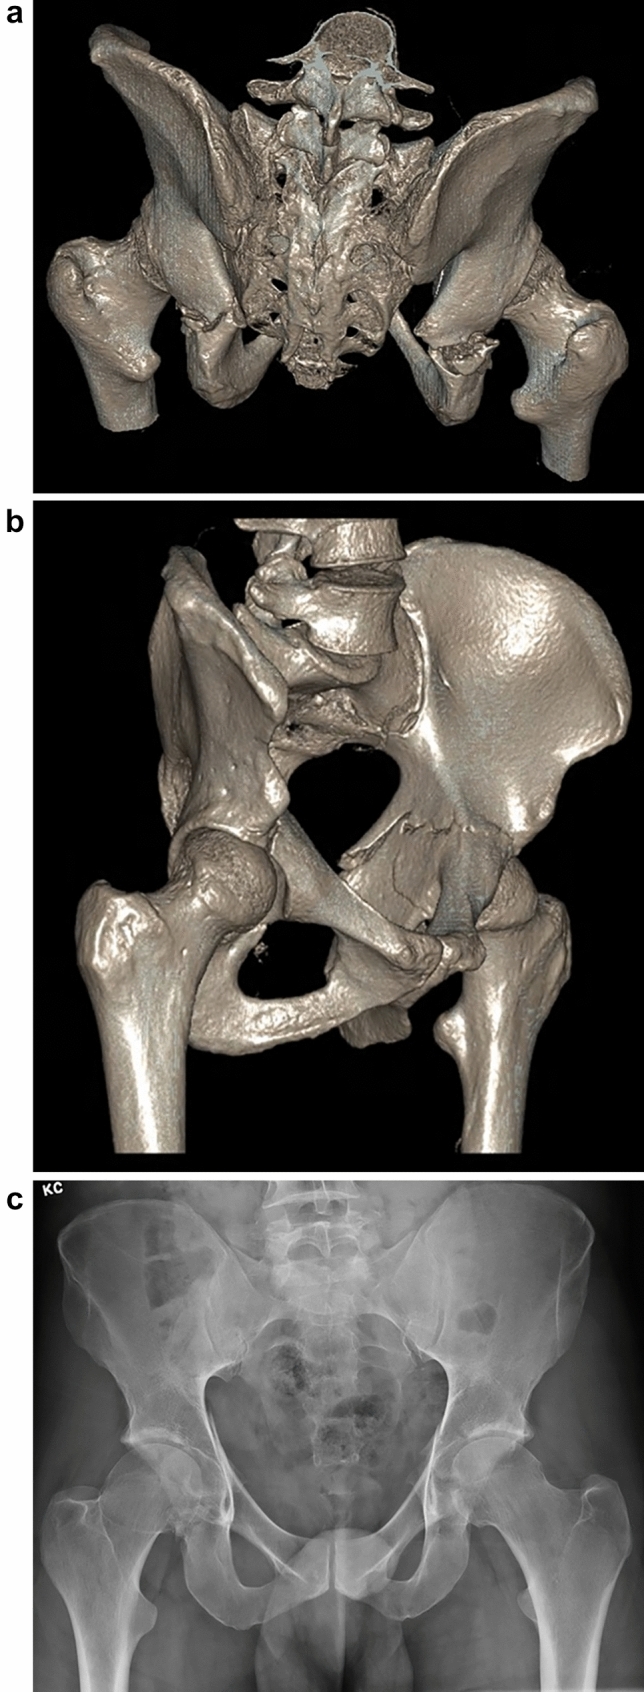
3D surface-rendered CT reconstruction images; posterosuperior view (a), right oblique view (b), demonstrating posterior displacement of the anterior ring and antero-posterior pelvis radiograph (c) demonstrating bilateral transverse acetabular fractures
